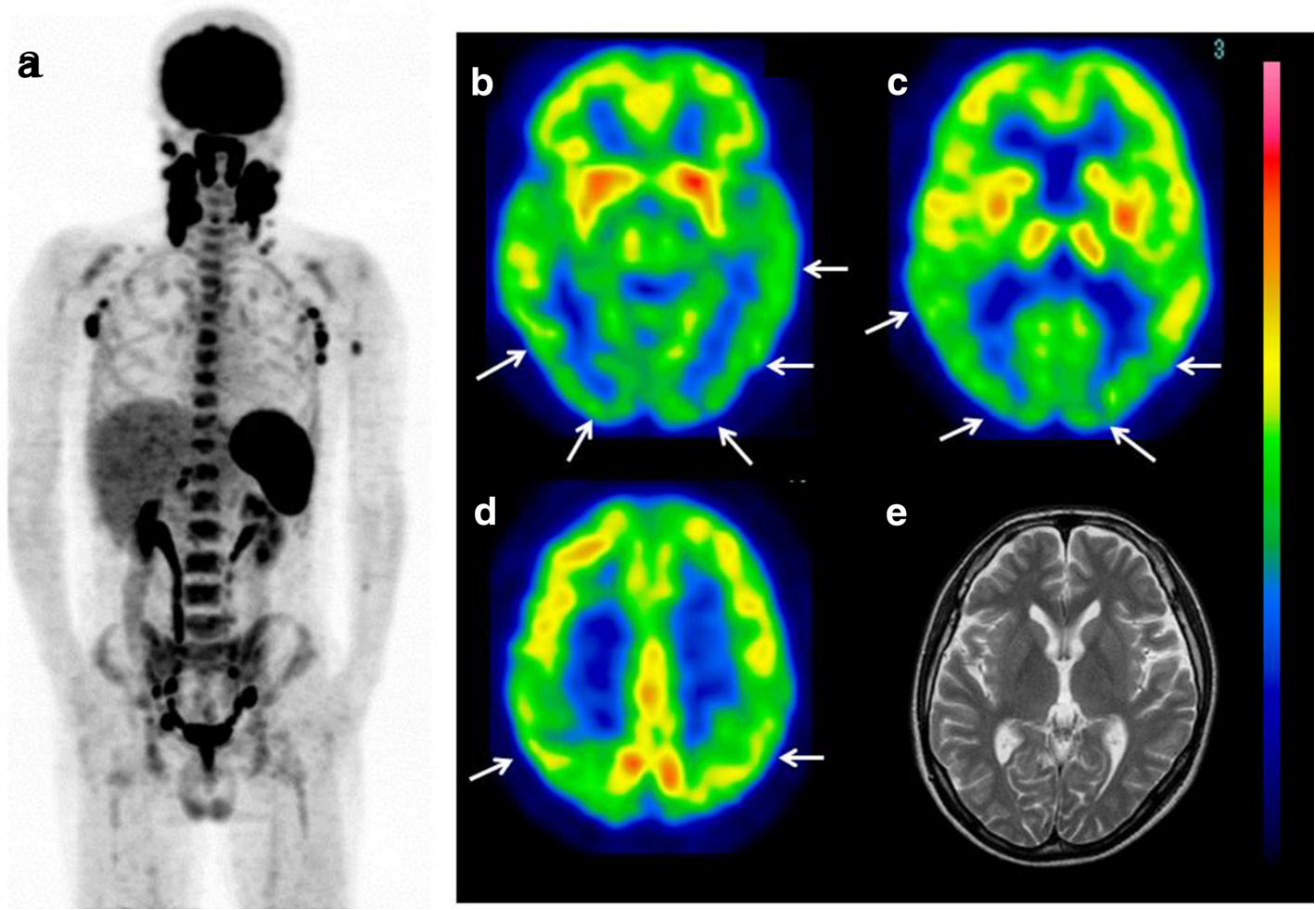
(Case 2: T-cell lymphoma). FDG-PET images of the whole body and brain. Whole-body FDG-PET showed a high uptake in the systemic lymph nodes, spleen and bone marrow (a). Brain FDG images demonstrated a reduction in glucose metabolism in the bilateral parieto-occipital regions (b–d). MRI showed no abnormalities in the brain (e)
